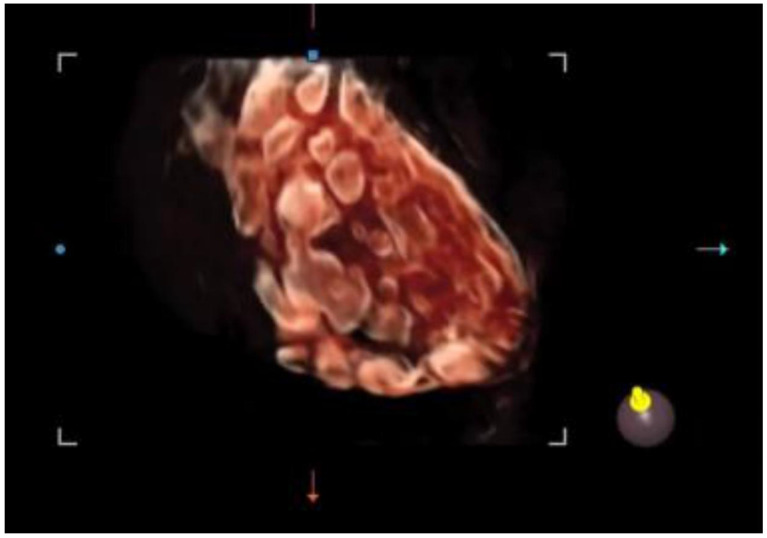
Three-dimensional ultrasound scan using the inversion mode.
